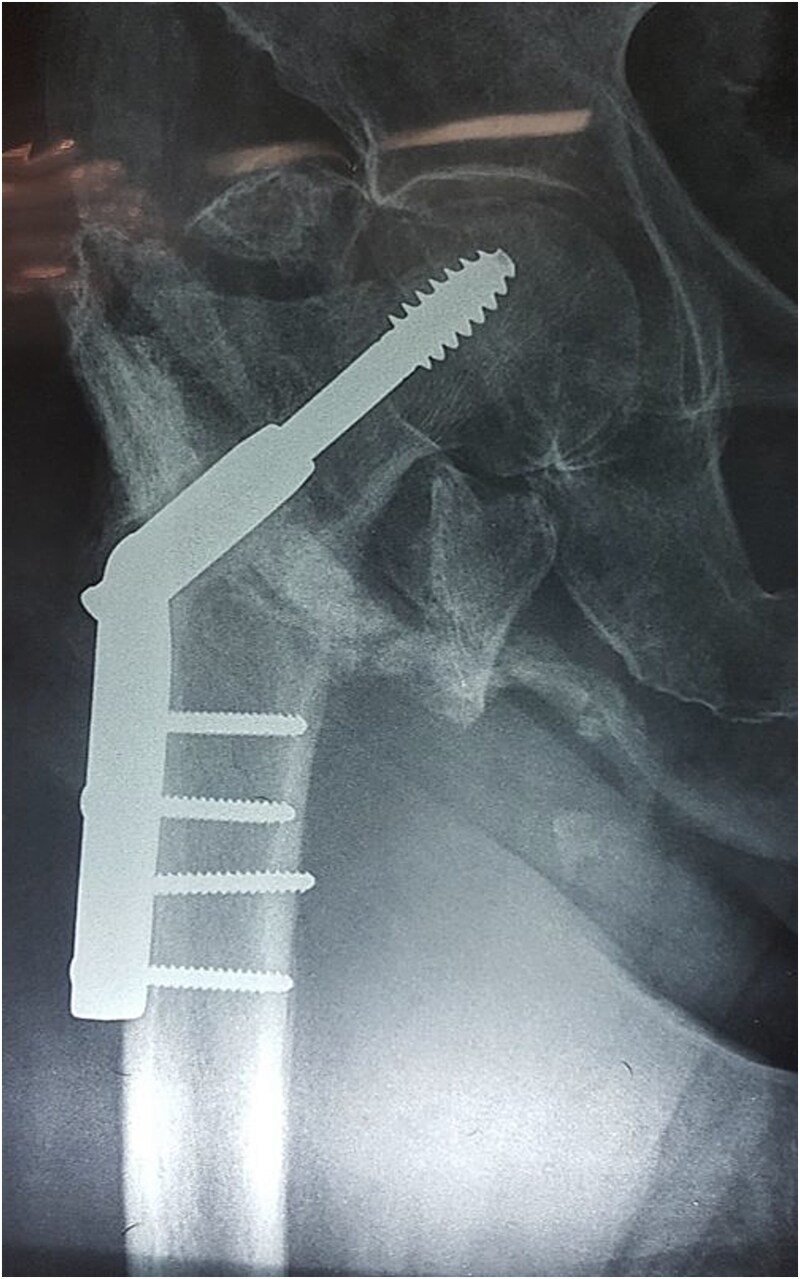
Plain radiograph of the right hip. Intertrochanteric femoral fracture previously treated with dynamic hip screw and plate fixation. Two cortical screws are visible protruding beyond the medial cortex of the femur, representing the probable mechanism of vascular injury.
